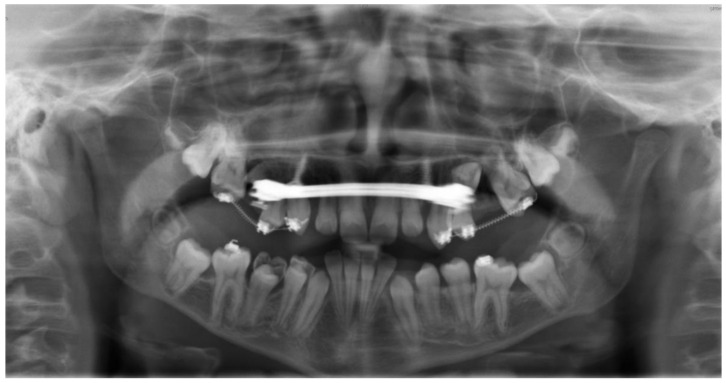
The follow-up panoramic radiograph at the age of 13 years reveals the displaced germs of teeth 15 and 25, partially superimposed by the cantilever structure in the palatal area. Both mini-implants show no sign of peri-implant osteolysis.
